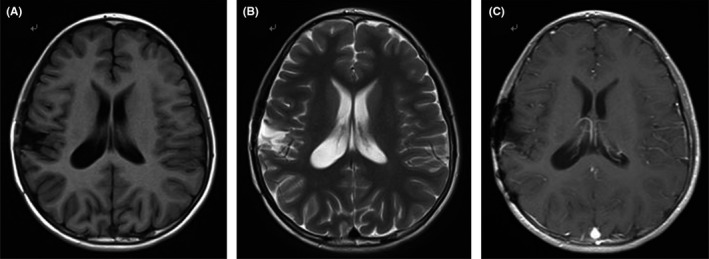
MRI after surgery showed partial cerebrospinal fluid retention in the right parietal lobe, T1‐weighted image (a), T2‐weighted image (b), enhanced T1WI (c)
